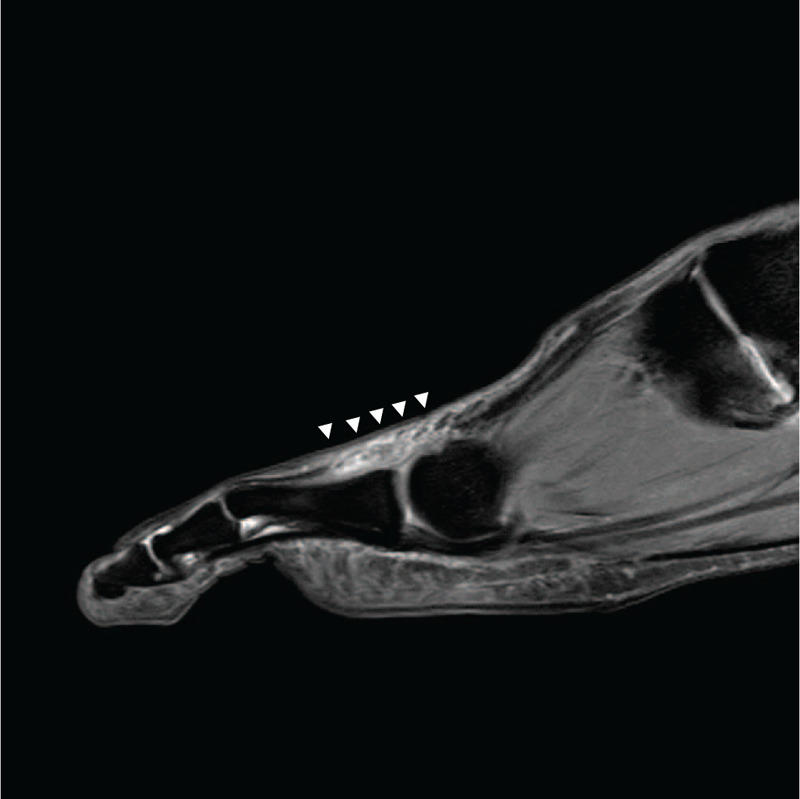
Preoperative T2-weighted sagittal magnetic resonance image showing ruptured second EDL with diffuse intratendinous heterogeneous signal change (white arrowheads).
